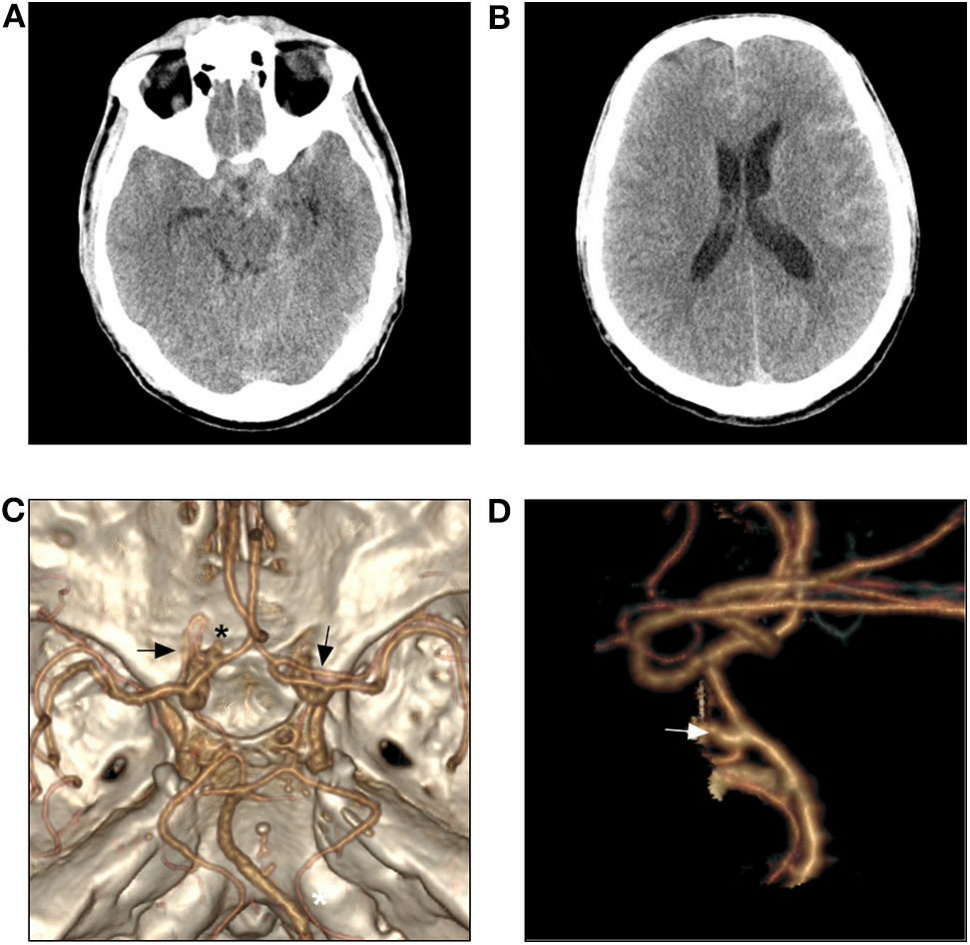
Head CT shows subtle SAH (A,B). CTA reveals two ac-MCAs originate, respectively from the A1 segment of the bilateral ACAs (C, black arrow) and an aneurysm originates from the beginning of the left ac-MCA (C,D, asterisk and white arrow). ACA, anterior cerebral artery; ac-MCA, accessory middle cerebral artery; CT, computed tomography; CTA, CT angiography.
